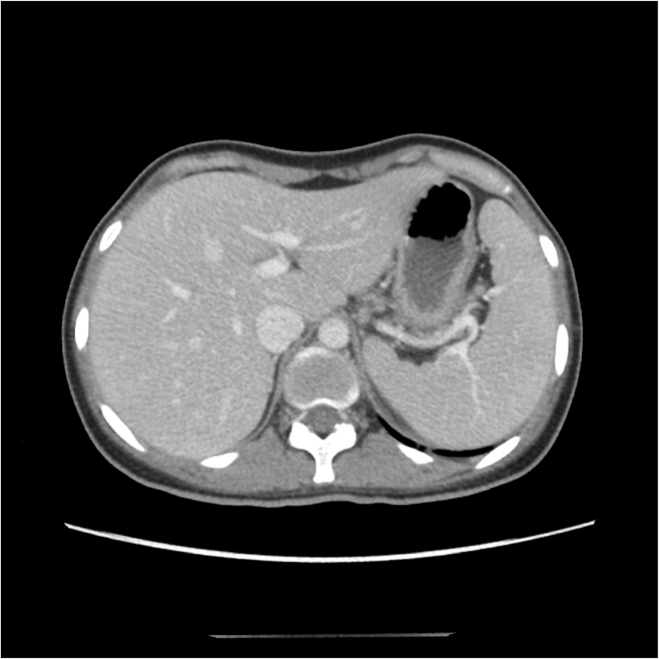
Computed tomography images showing splenomegaly.
